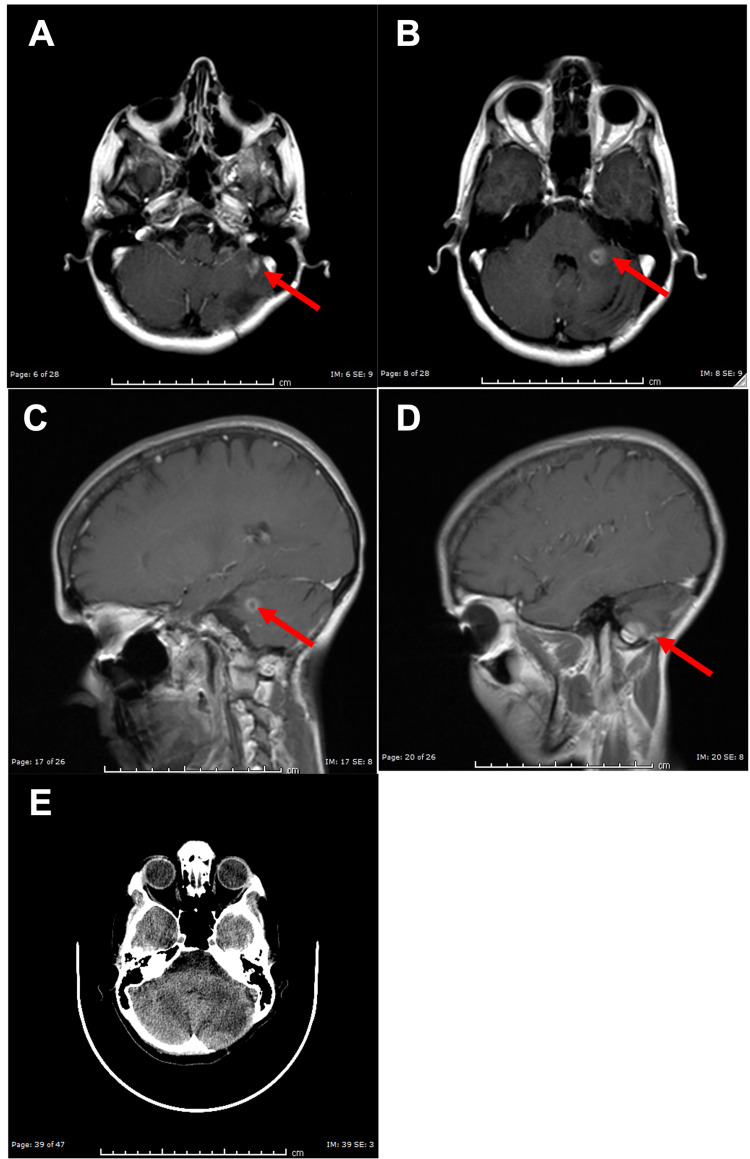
MR Images Following Final Dose of Stereotactic Radiosurgery (A, B) Axial T2-weighted MR images demonstrating new lesion within the brachium pontis (red arrow). (C, D) Sagittal T1-weighted MR images with gadolinium contrast demonstrating new lesion within the brachium pontis (red arrow). (E) (18F-FDG) PET with no abnormal uptake in the left cerebellum indicative of radiation necrosis. 18F-FDG: 2-deoxy-2-[fluorine-18] fluoro-D-glucose, PET: positron emission tomography.
